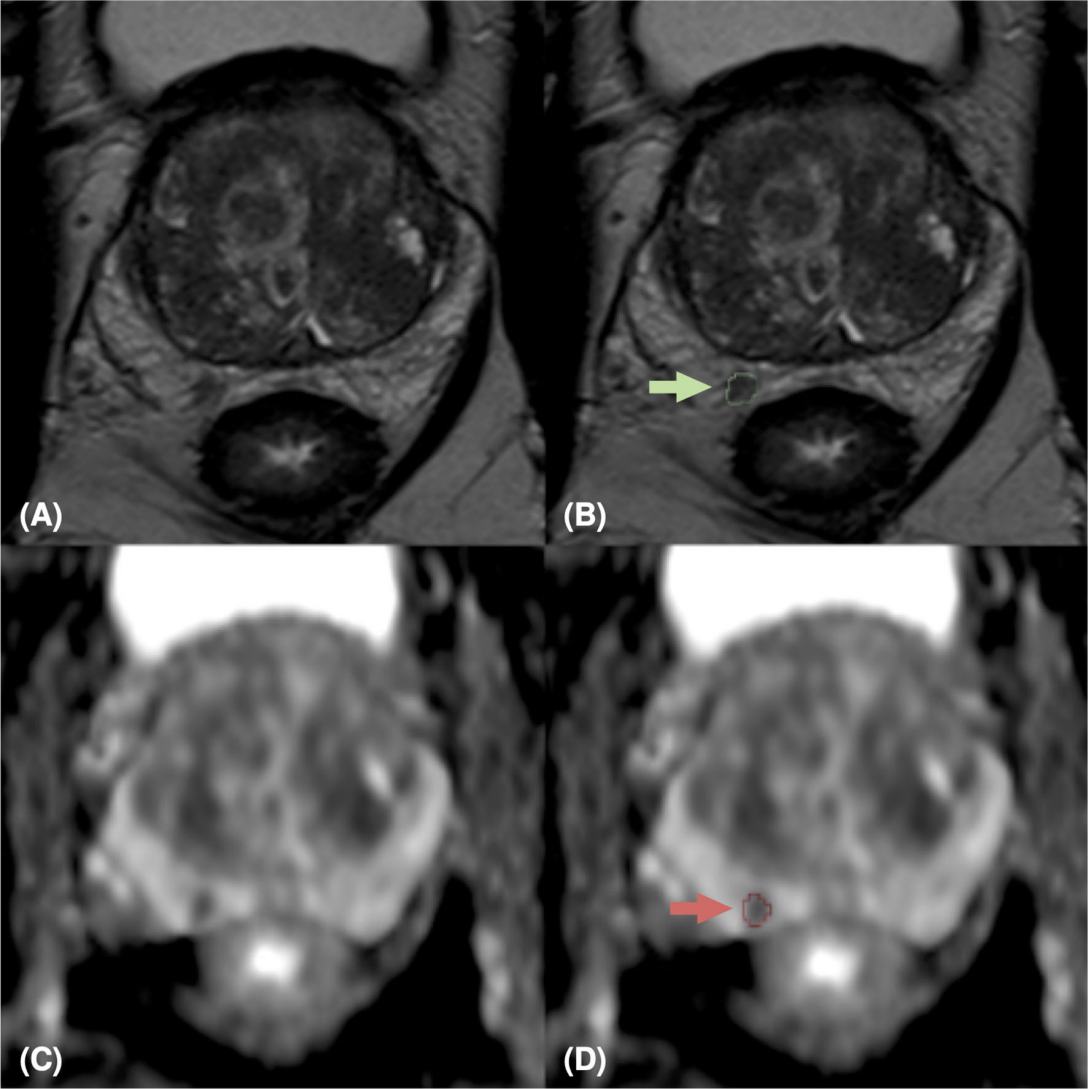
MpMRI of a 76-years old patient with indeterminate mpMRI result (PI-RADS=4), PSA=14 ng/ml, GS=4+4 (ISUP 4). MpMRI zoomed images containing the target lesion, respectively axial T2-weighted image (A), ADC map (C), and their relative lesion segmentations (B, D). The green (B) and red (D) arrows point out the segmented lesion in T2 and ADC images, respectively.
